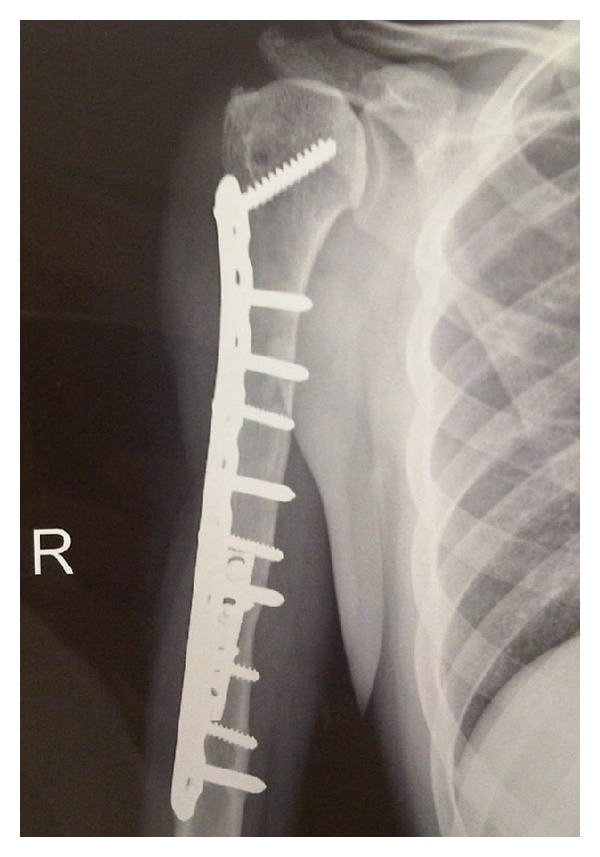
Anteroposterior radiograph of an osteoarticular allograft of the proximal humerus after 5 years of reconstruction.
